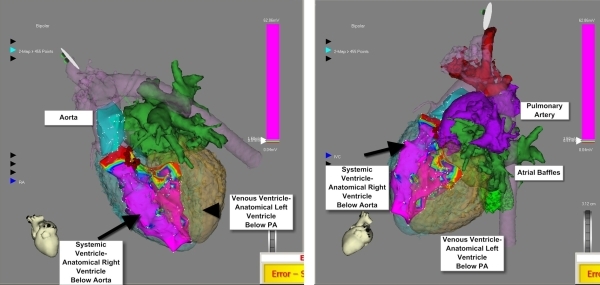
This image shows electroanatomical maps of the systemic ventricle and the ascending aorta integrated with the contrast enhanced CT image of the patient's heart. The image shows ablation lesions and the scarred interventricular septum of the heart.
